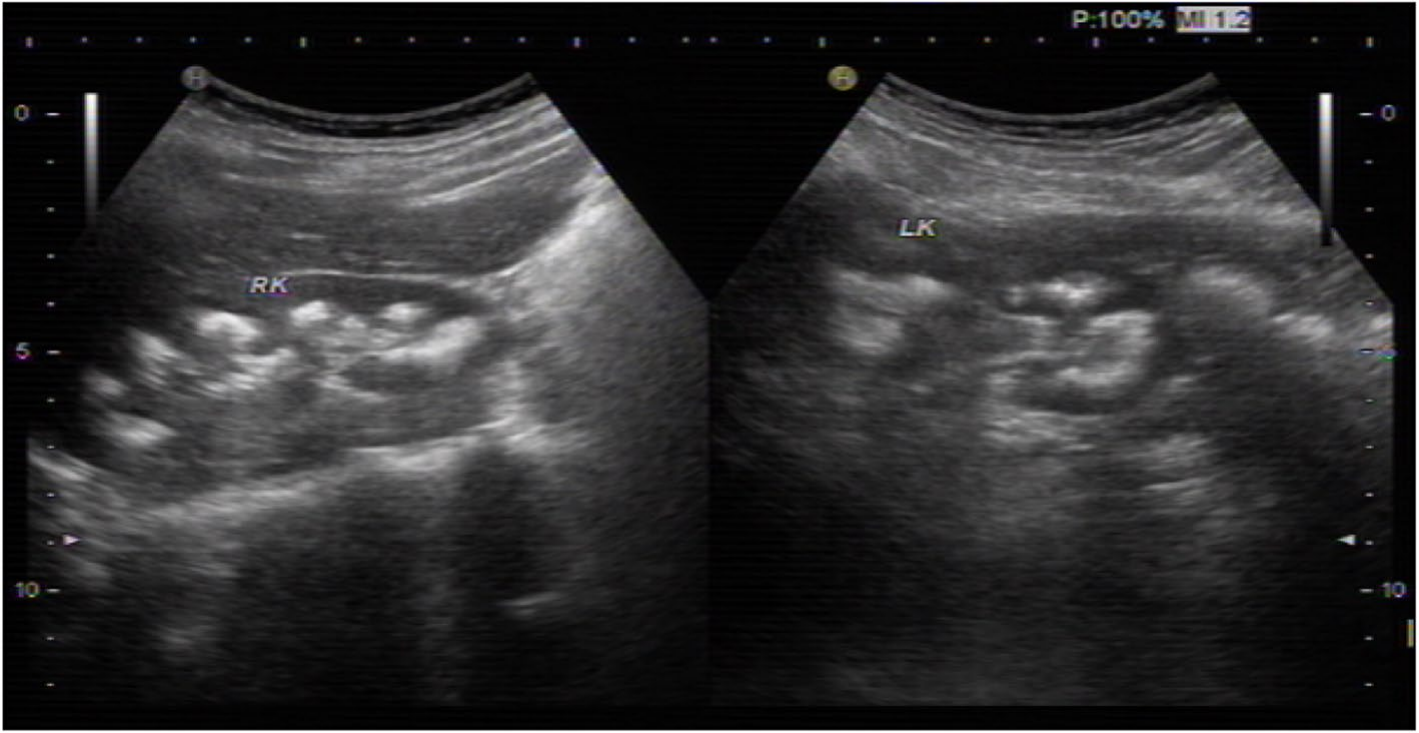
The urinary ultrasound showed enhanced echo of bilateral renal collecting system
